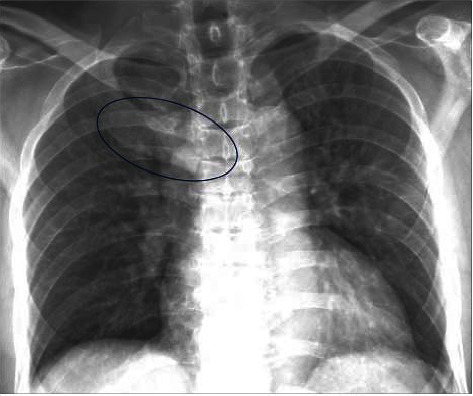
Posterior–anterior chest radiograph showing enlargement of the anterior right first rib and chondral cartilage with cortical thickening.
